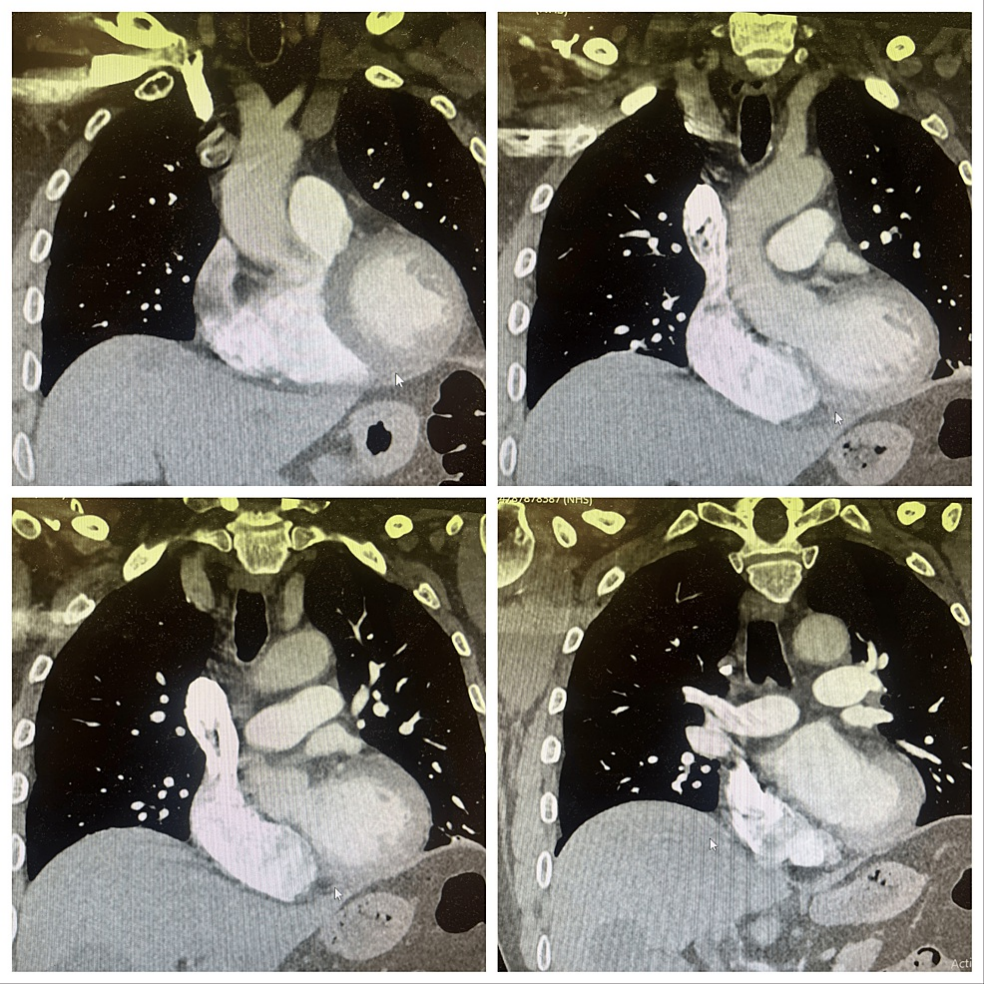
Cardiac magnetic resonance imaging (MRI) done to identify any infiltrative disease like amyloidosis/sarcoidosis
